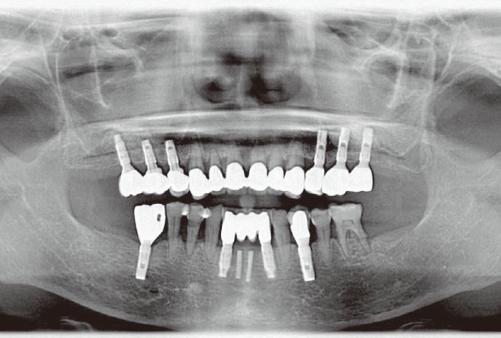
Postoperative panoramic radiograph following sinus re-augmentation along with the placement of dental implants.
